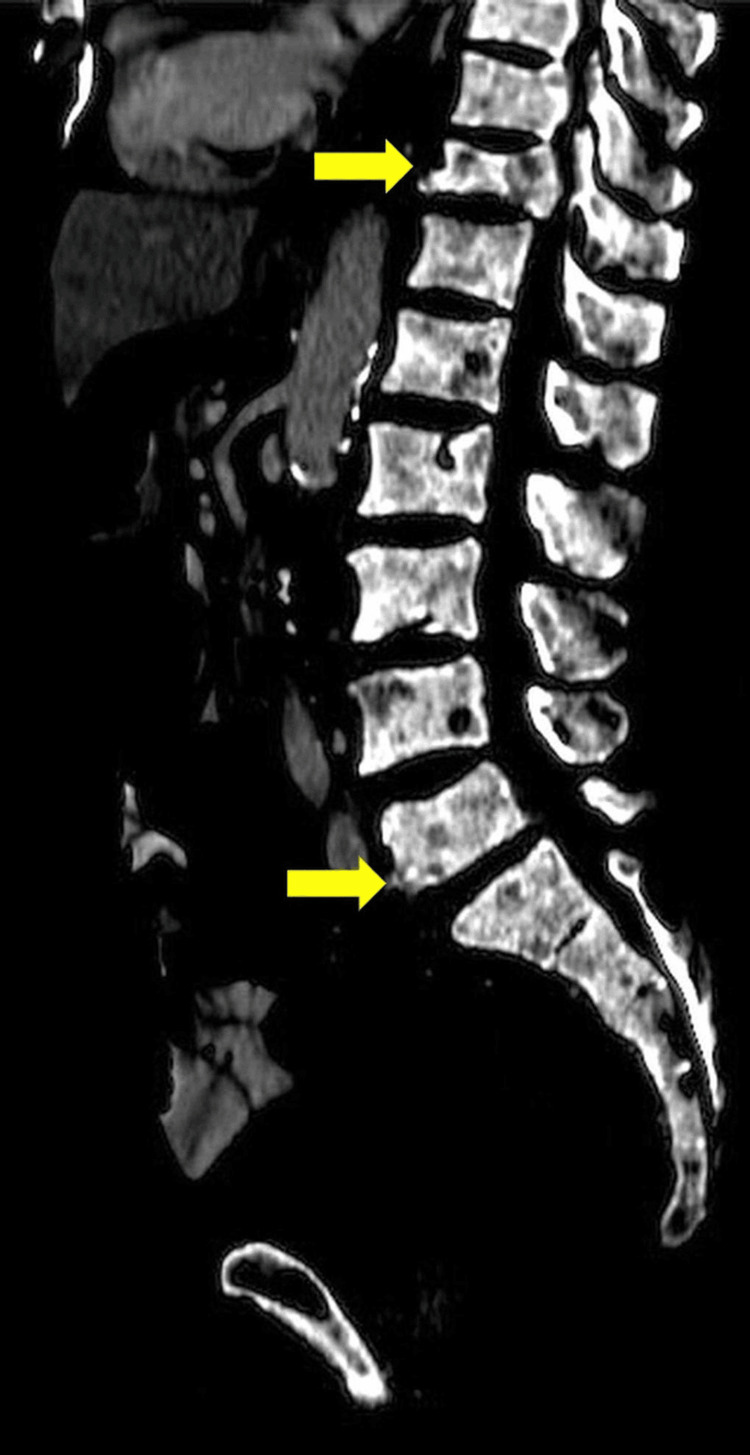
A sagittal CT scan of the whole abdomen demonstrated diffuse mixed osteoblastic and lytic lesions involving multiple bony structures, along with the collapse of the T11 vertebra (upper yellow arrow) and the L5 vertebra (lower yellow arrow) CT: computed tomography
